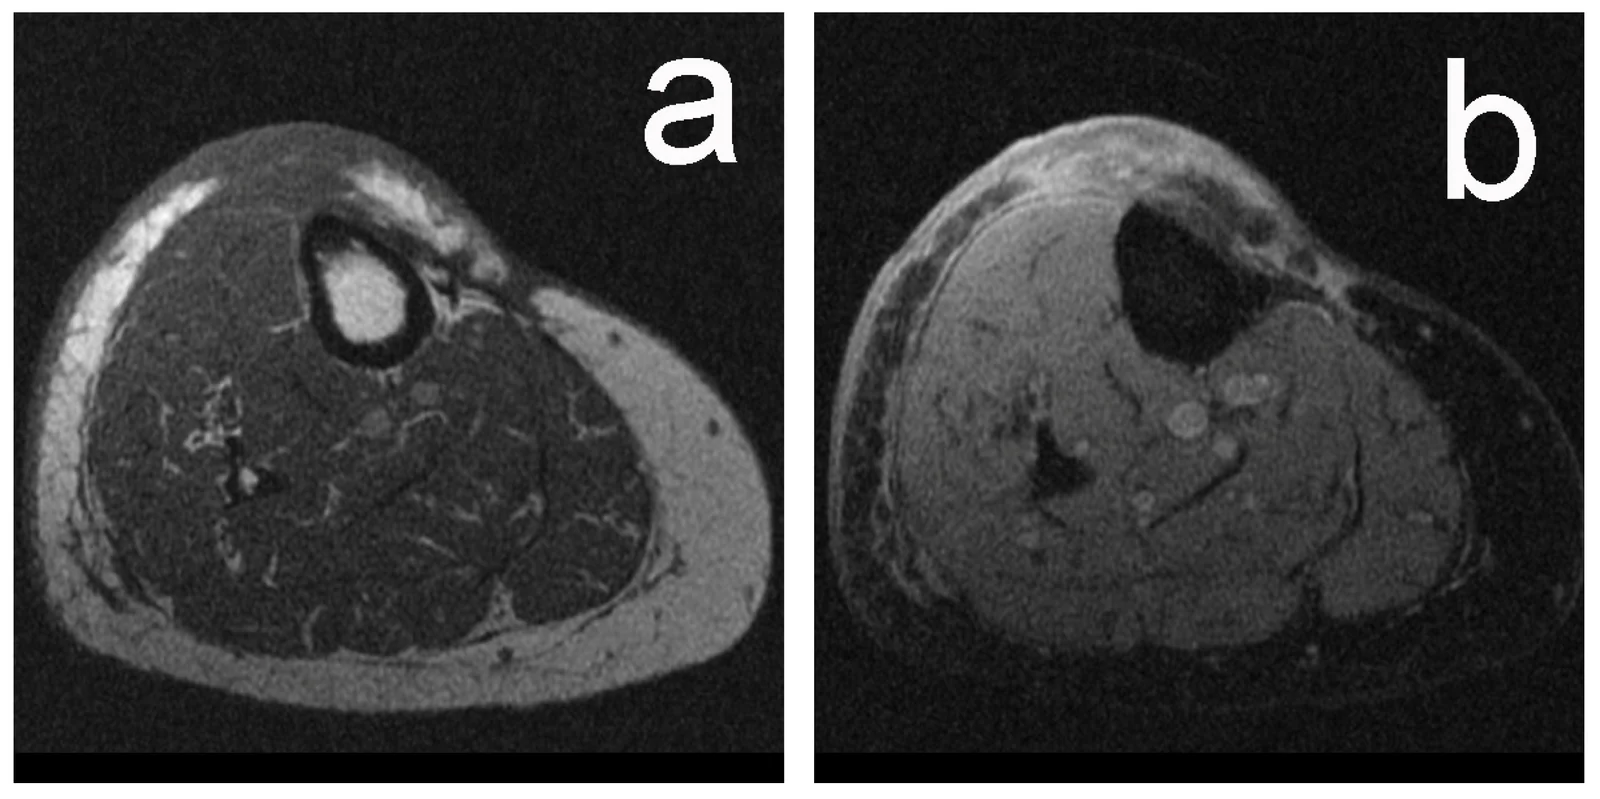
Preoperative contrast-enhanced MRI (a,b) of the right leg demonstrating the recurrent soft-tissue lesion extending through the anterior soft tissues to the anterior surface of the proximal tibia.
